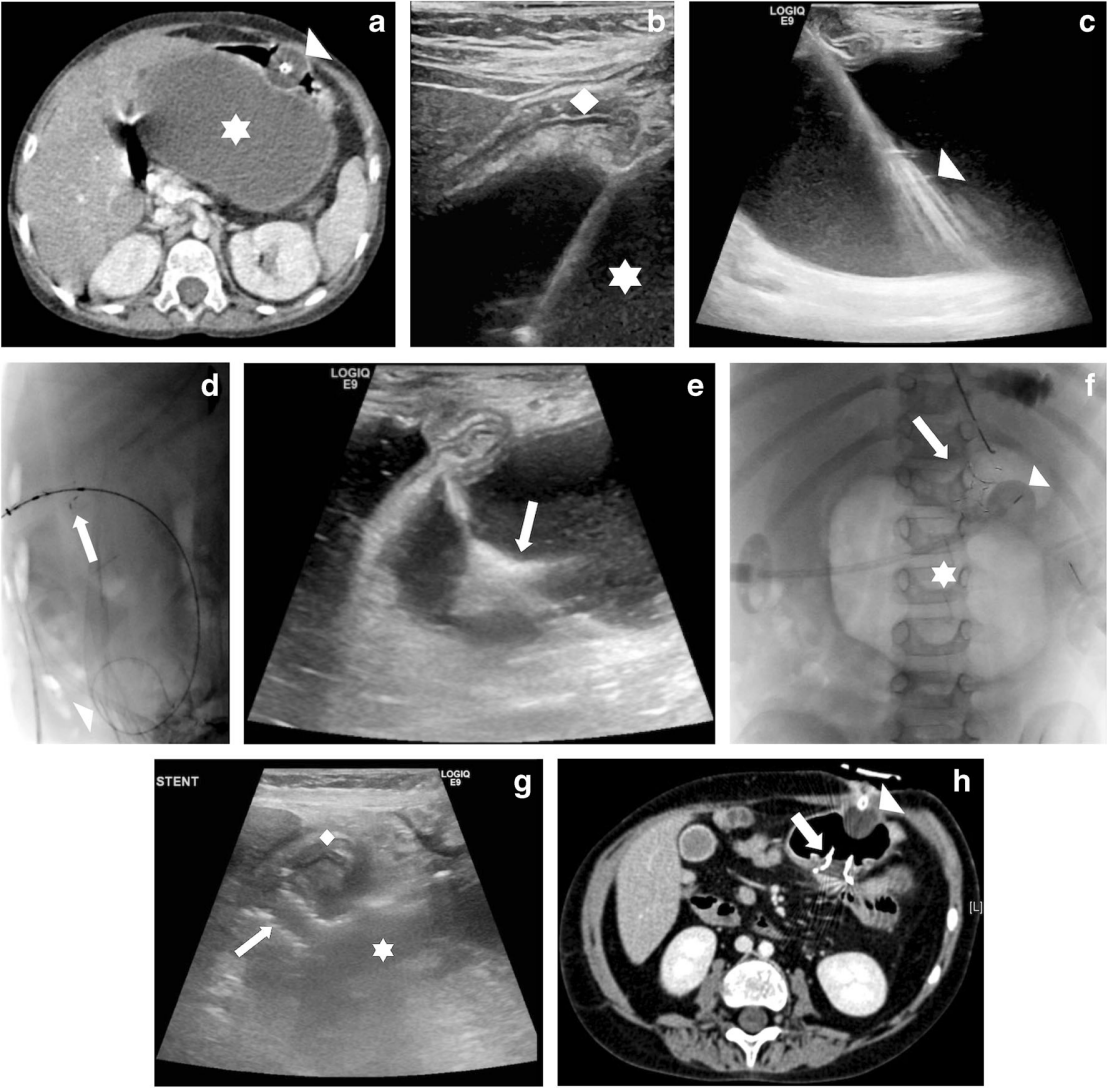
Pancreatic pseudocyst in a 6-year-old girl (Table 1, patient 1). a Contrast-enhanced axial CT image demonstrates a large pancreatic pseudocyst (*star*) and compressed stomach with balloon-retained gastrostomy (*arrowhead*). b Sagittal US image demonstrates puncture of the pseudocyst (s*tar*) through the stomach (*diamond*). c Sagittal US image demonstrates guidewire insertion and direction to deep component of pseudocyst, where it was coiled (*arrowhead*). d Lateral fluoroscopic image demonstrates coiled wire (*arrowhead*) and the start of stent deployment with flaring of distal end (*arrow*). e Sagittal US image confirms the distal flange flaring in the pseudocyst (*arrow*). f Anteroposterior fluoroscopic image demonstrates final stent position *(arrow)*. There is gas in the pseudocyst (*star*). A balloon-retained gastrostomy is in situ *(arrowhead).*g Sagittal US image day 1 post-procedure demonstrates stent position (*arrow*). The stomach *(diamond)* is empty and the pseudocyst (*star*) is smaller than before the procedure. h Contrast-enhanced axial CT image 1 month after stent insertion demonstrates the stent (*arrow*) and resolution of the pseudocyst. A balloon-retained gastrostomy is present (*arrowhead*)
